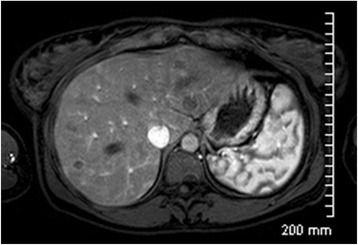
T1-weighted contrast-enhanced axial MR image four weeks after TAE showing a reduction in the volume of enhancing hepatic metastases
